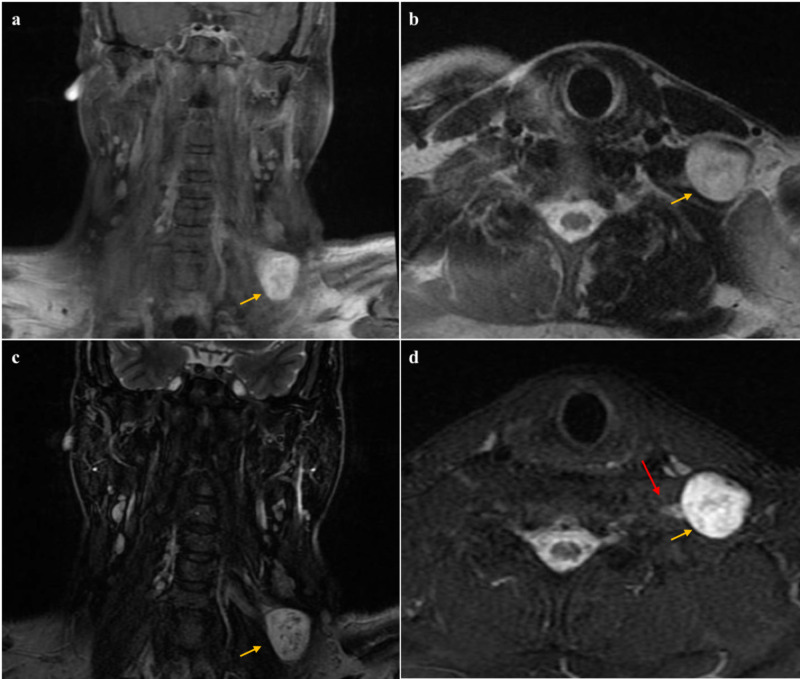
MRI images of the patient's neck The yellow arrow indicates the tumor; a and b: T1-weighted images, coronal and axial respectively, showing heterogenous hyperintensity of the tumor; c and d: T2-weighted images, coronal and axial, showing hyperintensity of the tumor and increased intensity of C6 nerve root (red arrow) MRI: magnetic resonance imaging
